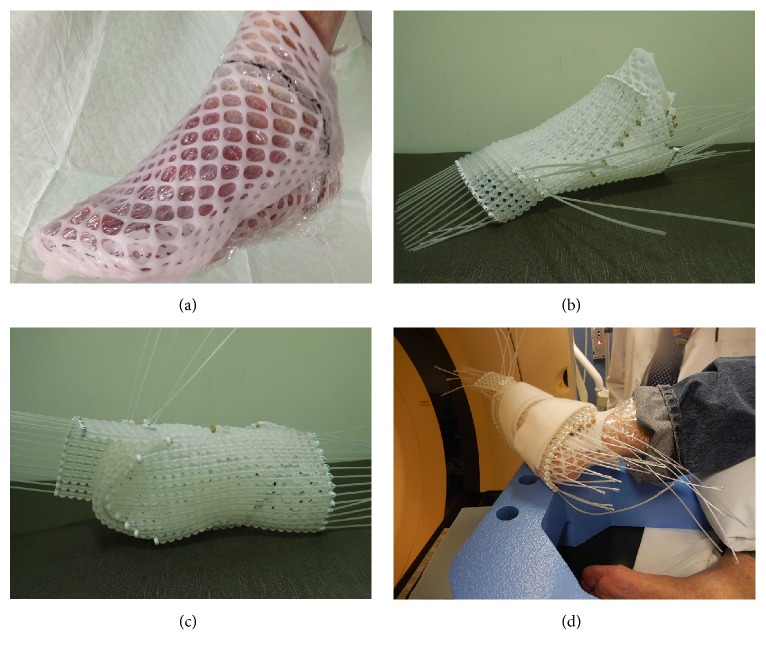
(a) Two-part thermoplastic mesh encompassing the left foot with proximal border of treatment area outlined in black. (b) Complete Freiburg device consisting of a total of 39 catheters. (c) Plantar aspect of the Freiburg device. (d) Positioning of the left foot for CT scan and treatment.
